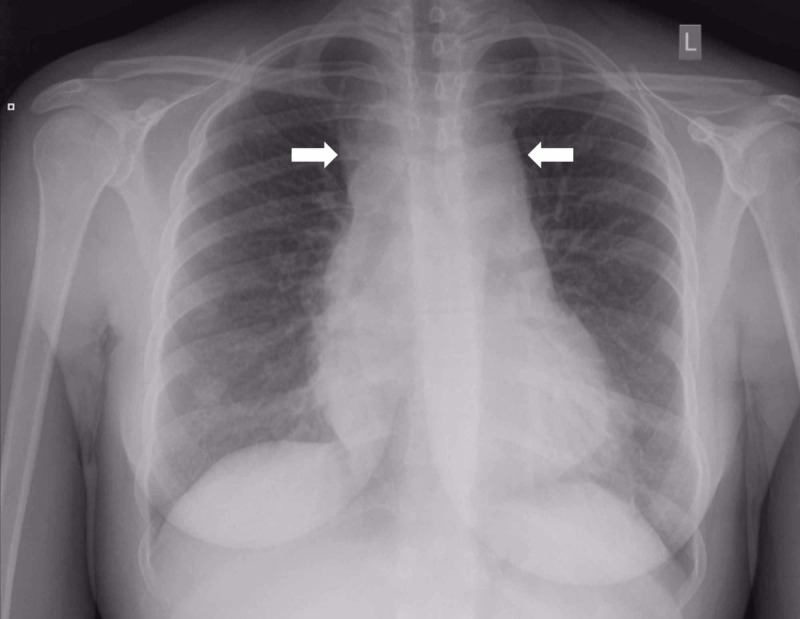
Plain film of the chest demonstrating a widening mediastinal silhouette (white arrows).
